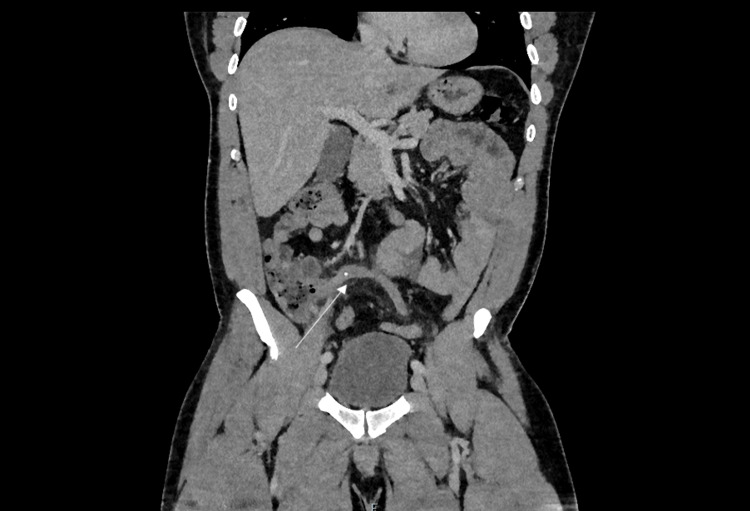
CT abdomen/pelvis showing a dilated, fluid-filled appendix with an appendicolith at the proximal aspect in keeping with acute appendicitis.
